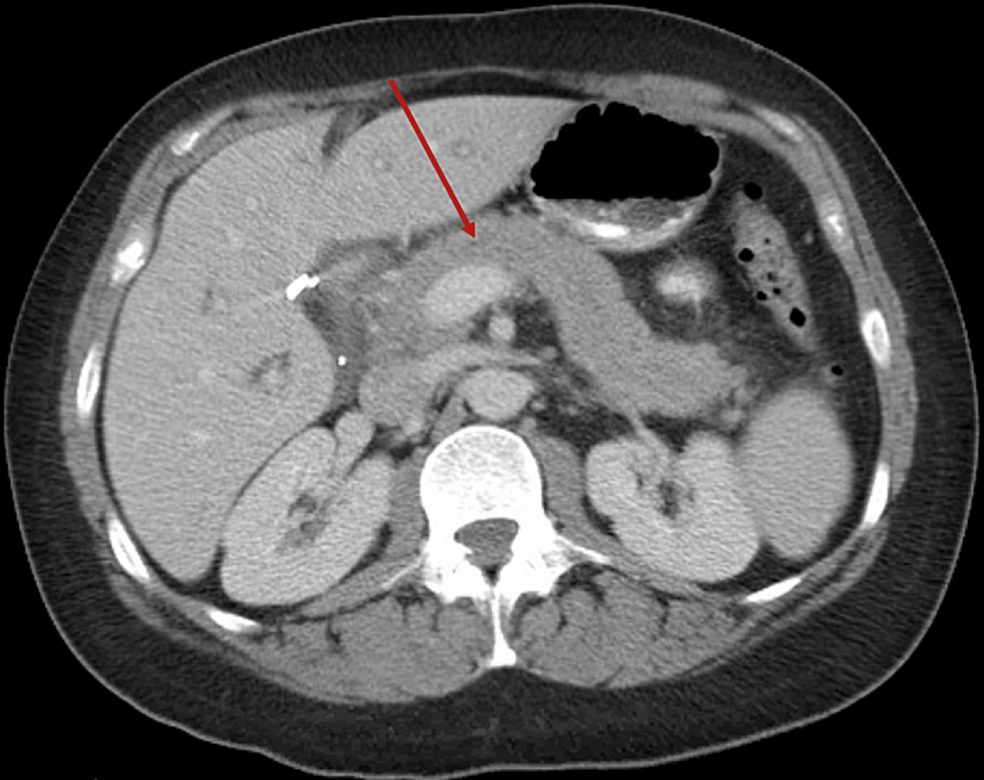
Computed tomography (CT) imaging of abdomen/pelvis, transverse section, showing inflammation of the pancreas and surrounding area consistent with pancreatitis (red arrow).
